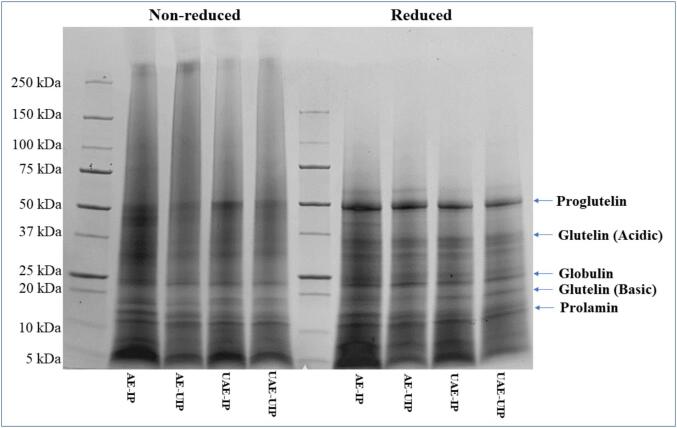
SDS-PAGE of extracted RBPs (AE-IP: Alkaline extraction with isoelectric precipitation; AE-UIP: alkaline extraction with ultrasound-assisted isoelectric precipitation; UAE-IP: ultrasound-assisted alkali extraction with isoelectric precipitation; and UAE-UIP: ultrasound-assisted alkali extraction with ultrasound-assisted isoelectric precipitation).
